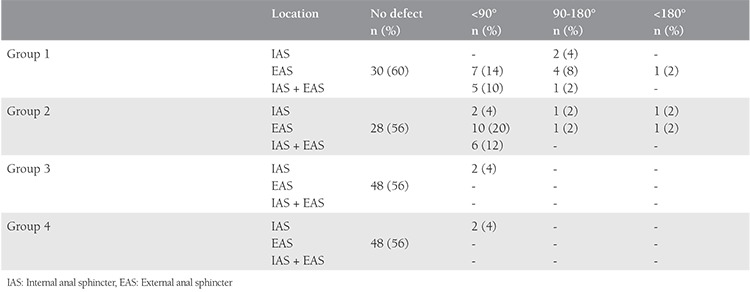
Endoanal ultrasound data of participants that location and size of defect at all groups
